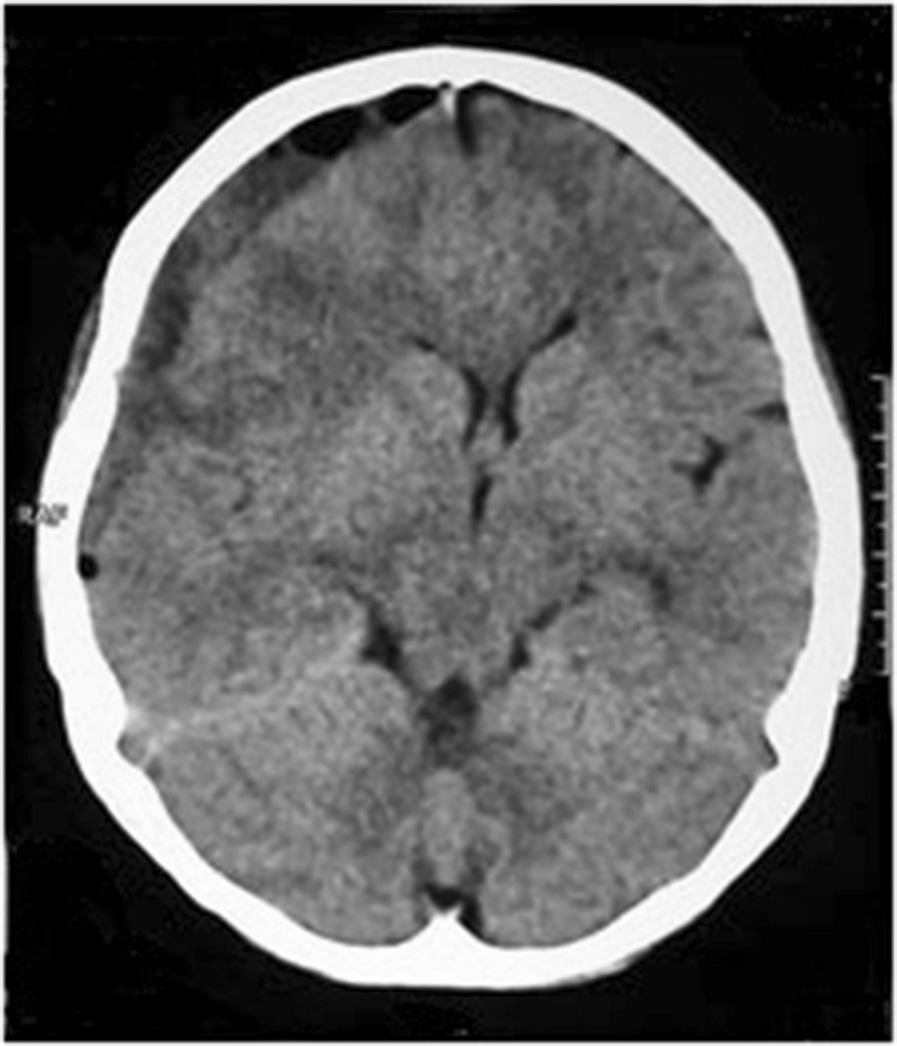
Image of computed tomography scan of brain on postpartum day 39: after the burr-hole aspiration of subdural haemorrhage.
